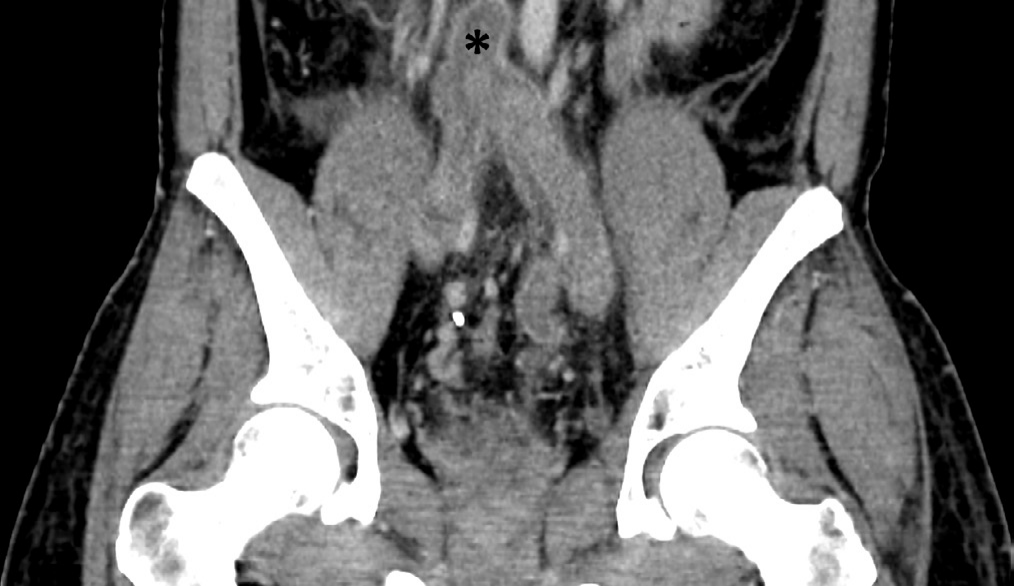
Contrast-enhanced CT, coronal reformat, showing the thrombus in the Inferior Vena Cava cephalad to the iliac veins converging (asterisk).
